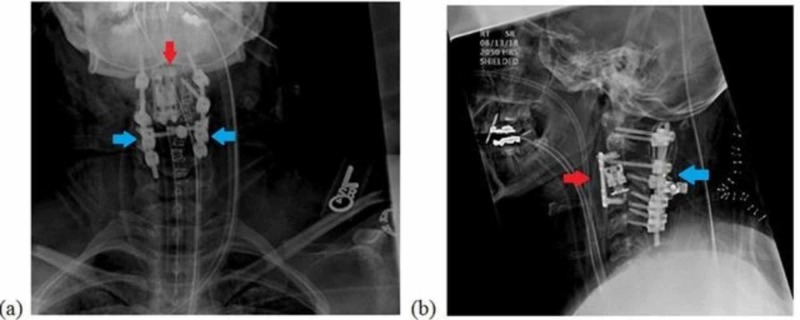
Postoperative cervical radiographs: (a) AP view and (b) lateral view with red arrows show a 12 mm vertebral body replacement cage was placed and a 26 mm anterior plate and blue arrows showing posterior instrumentation of C1-C4 with sublaminar wiring between C1 and C2.
